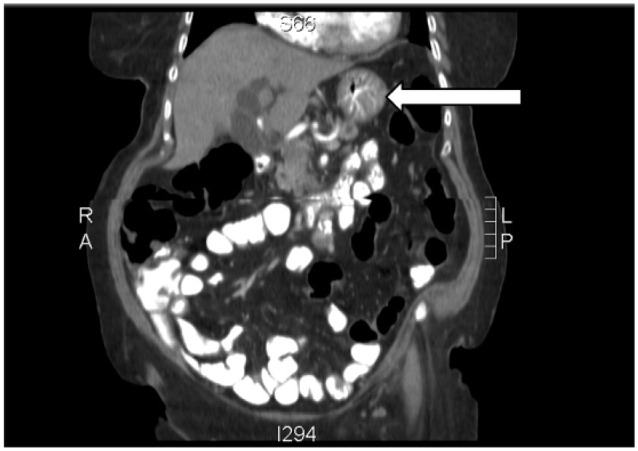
CT scan image of Case 1.
